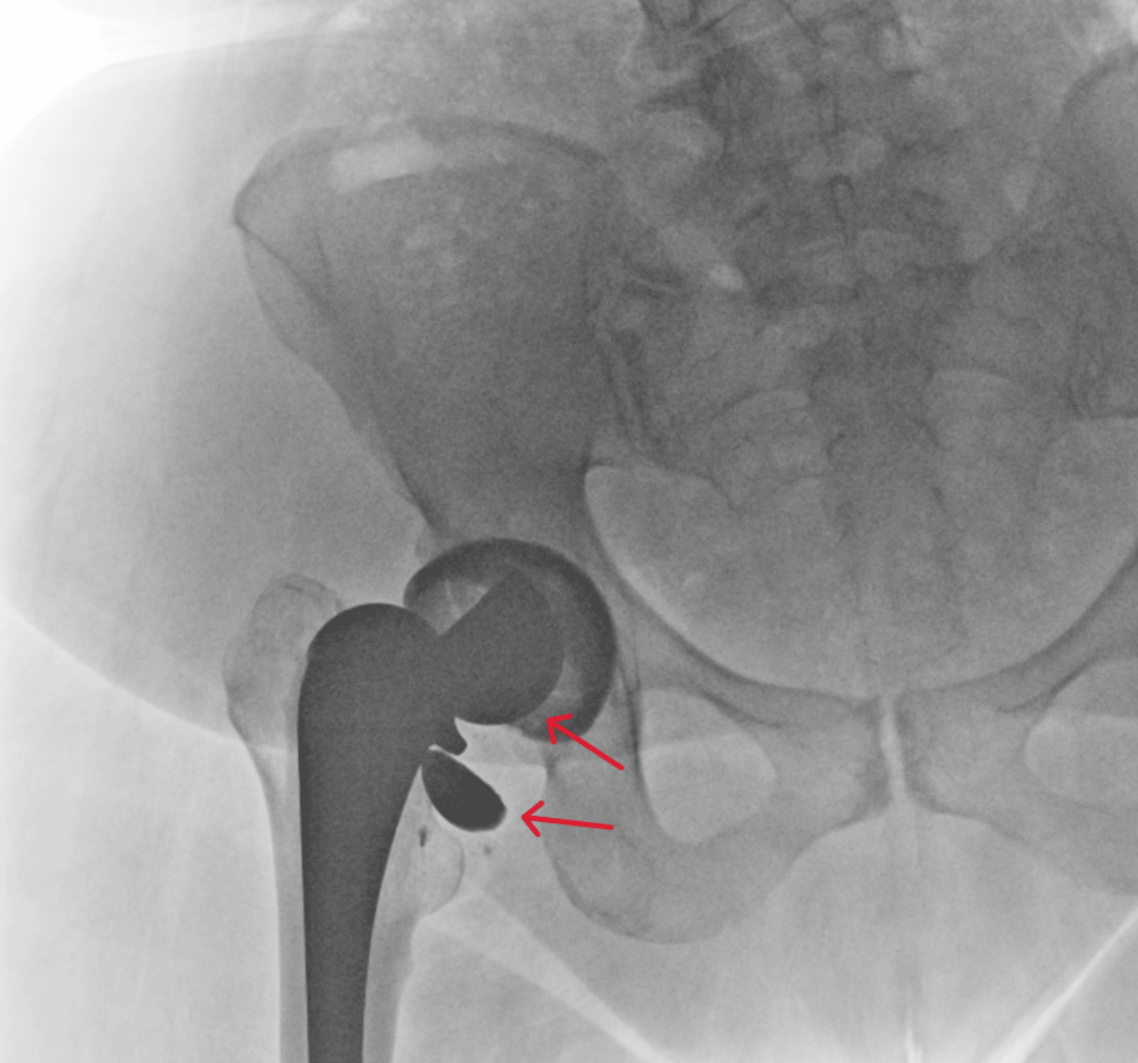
Anterior radiograph of the pelvis showing ceramic head fracture on the right side. Ceramic head fragments are identified by arrows.
